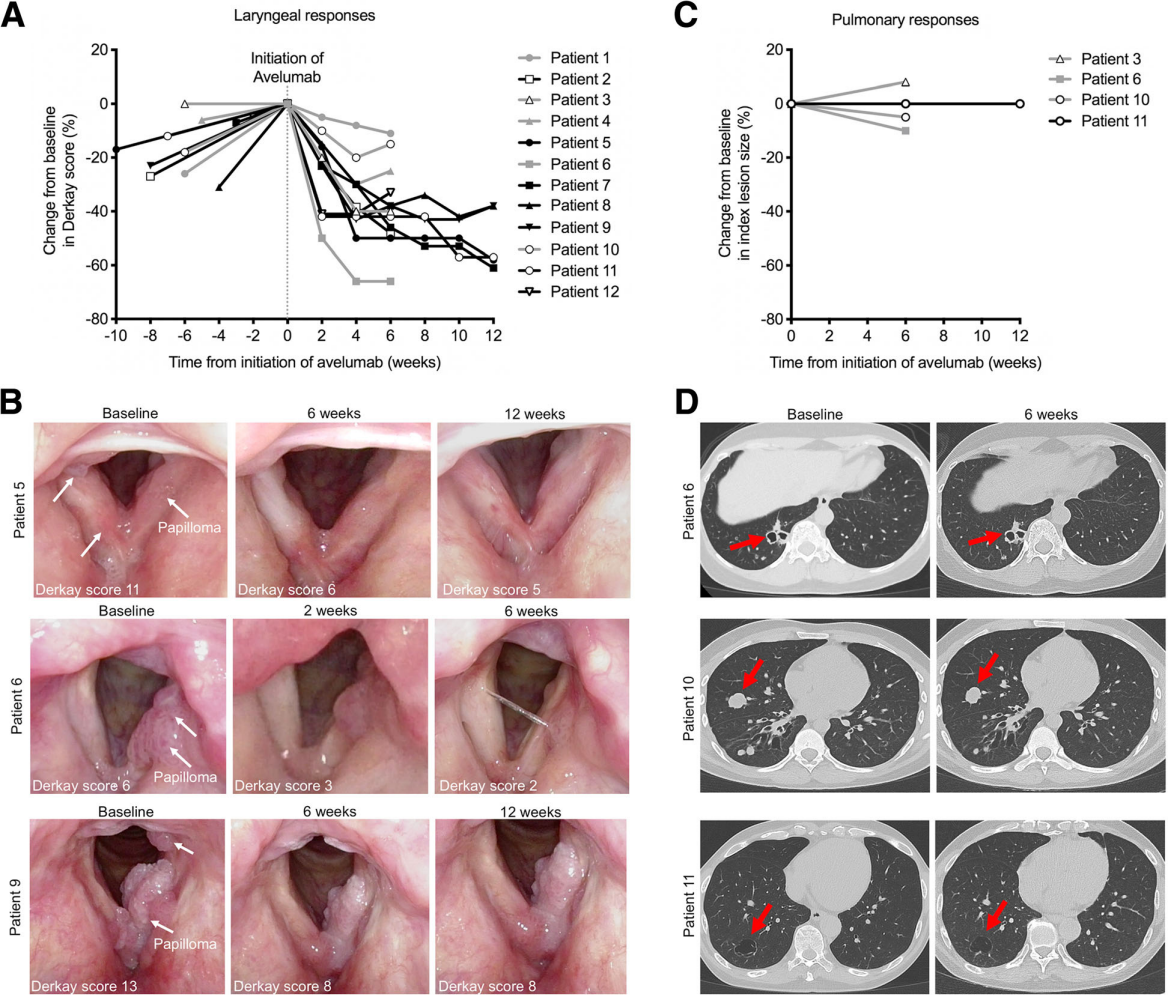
Clinical response following initiation of avelumab in patients with recurrent respiratory papillomatosis. a, A spider plot of change in laryngeal disease burden for each patient, as measured by anatomic Derkay score, is shown. The dotted line shows the time that avelumab was initiated. The negative time points reflect screening assessments prior to starting treatment. Patients 5, 7, 8, 9 and 11 received 6 doses of avelumab over 12 weeks. Patients 1, 2, 3, 4, 6, 10 and 12 received three doses over 6 weeks. b, Representative endoscopic images of RRP lesions for patients 5, 6 and 9 at the timepoints indicated are displayed. c, A spider plot of the change in pulmonary disease burden for each patient, as measured by CT scan imaging per RECIST1.1 guidelines, is shown. d, Representative images from CT scans from patients 6, 10 and 11 are displayed. Red arrows point to index lesions
